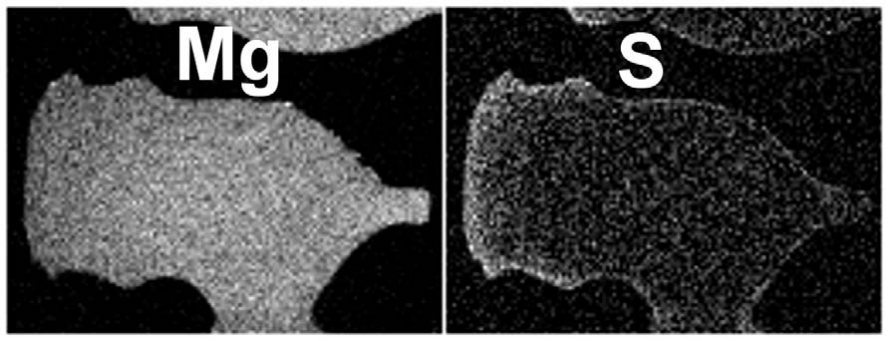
Maps of X-ray intensities. Intensities of Mg (left) and S (right) detected across the area used for chemical analyses. In both maps, brightest regions are those with highest abundance.
